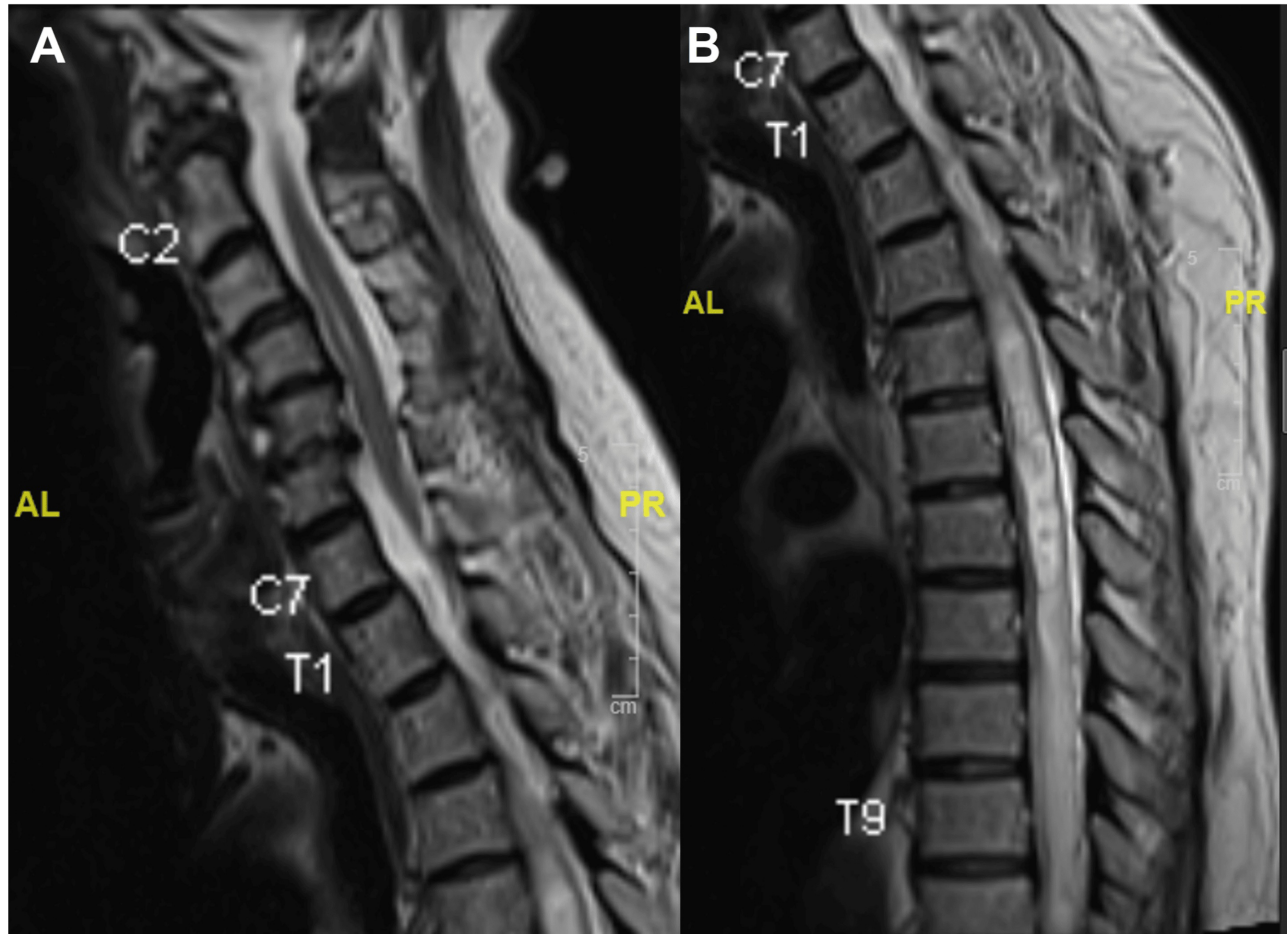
T2-weighted imaging of the MRI whole spine demonstrating diffuse syrinx along the entire spinal cord with associated cord expansion
